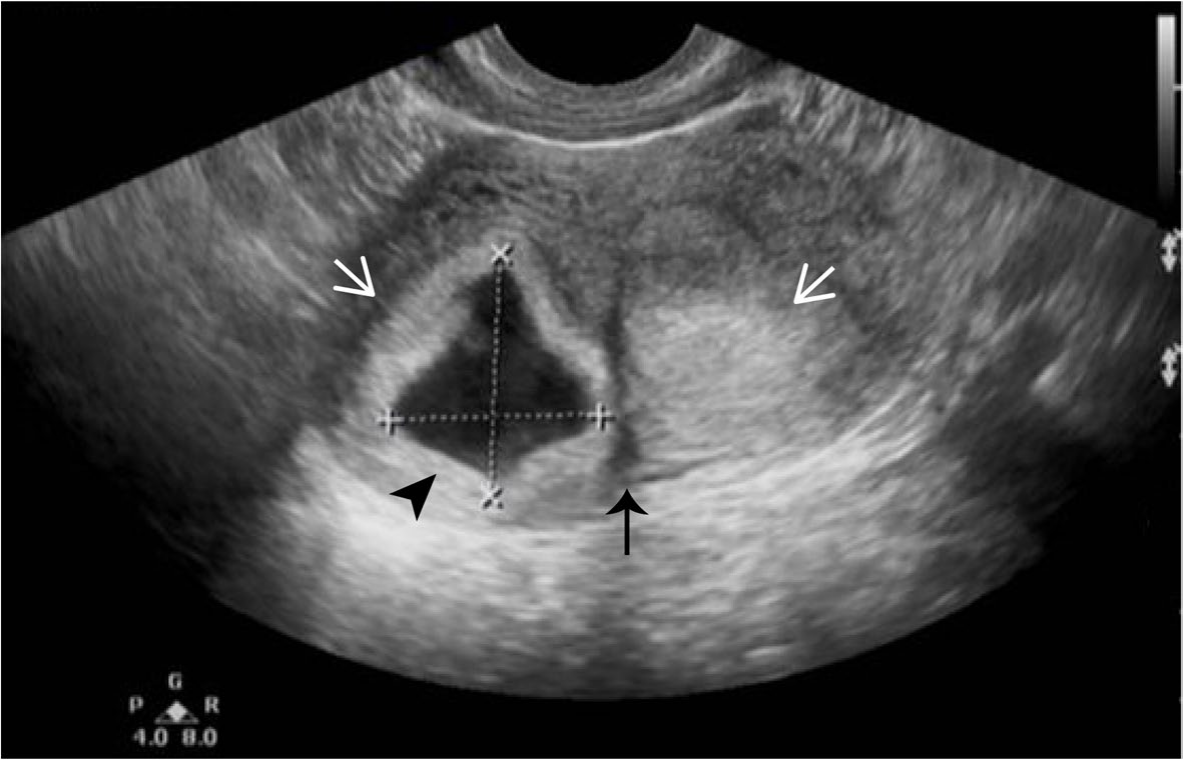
Two-dimensional ultrasound images during the first trimester. A uterine septum (black arrow) divides the uterus into two hemicavities (white arrows). Pregnancy occurs in the right blind hemicavity (black arrow head)
